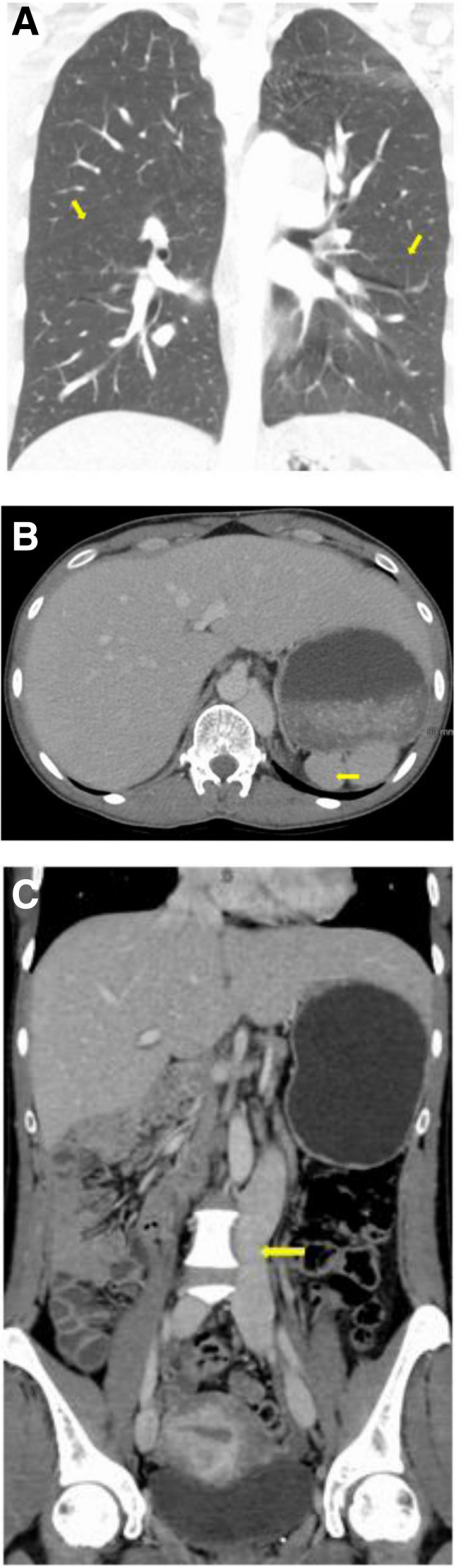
A. CT scan of the chest with contrast. Coronal section showing bilateral bilobed lungs (yellow arrows depict the major fissure). B. CT scan of the abdomen. The arrow points to multiple splenic lobules suggestive of polysplenia. C. CT scan of the abdomen. Coronal section showing azygous continuation of inferior vena cava
